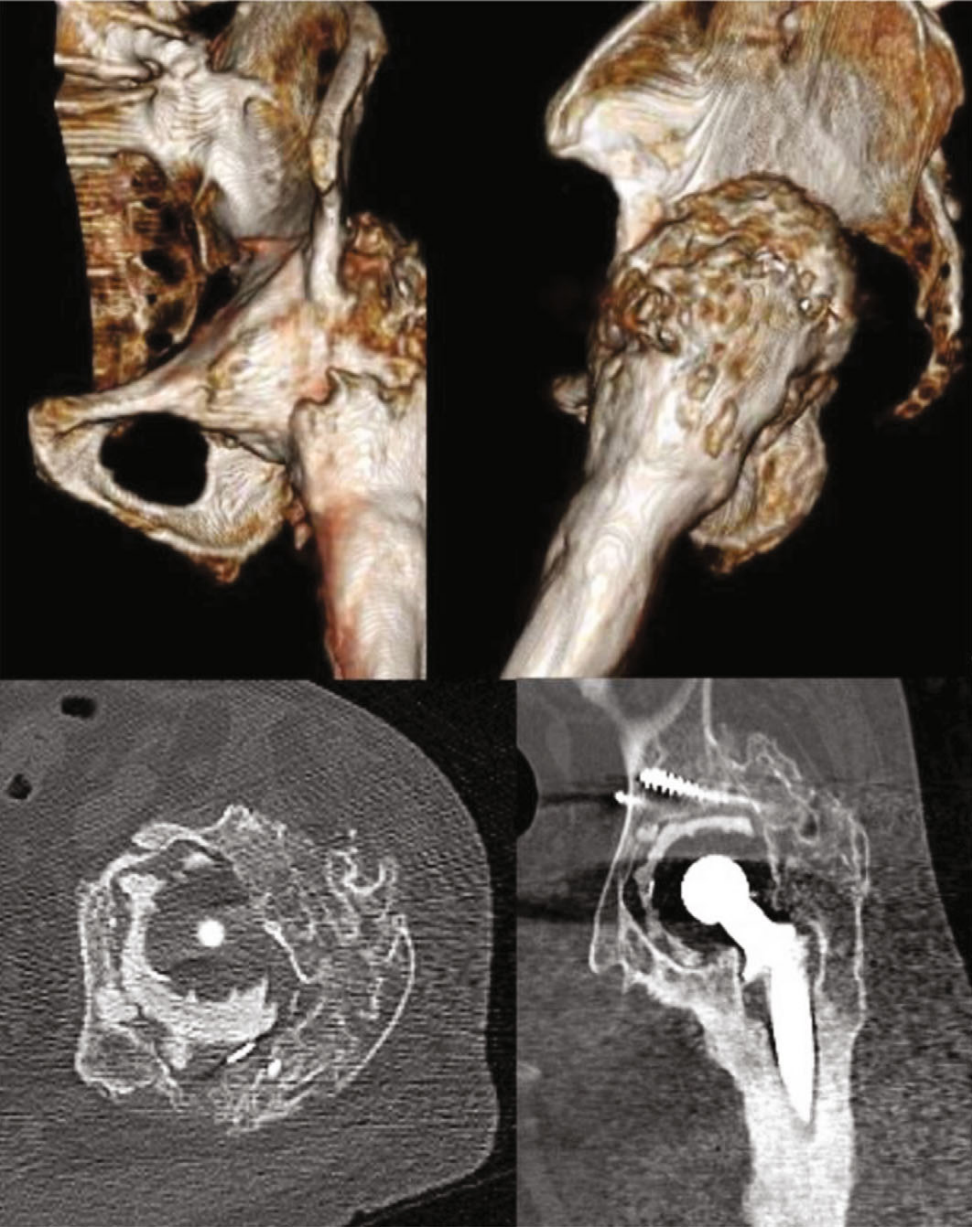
Three-dimensional computed tomography images showing severe heterotopic ossification around the hip joint and loosening of the acetabular cup in a 78-year-old man.
